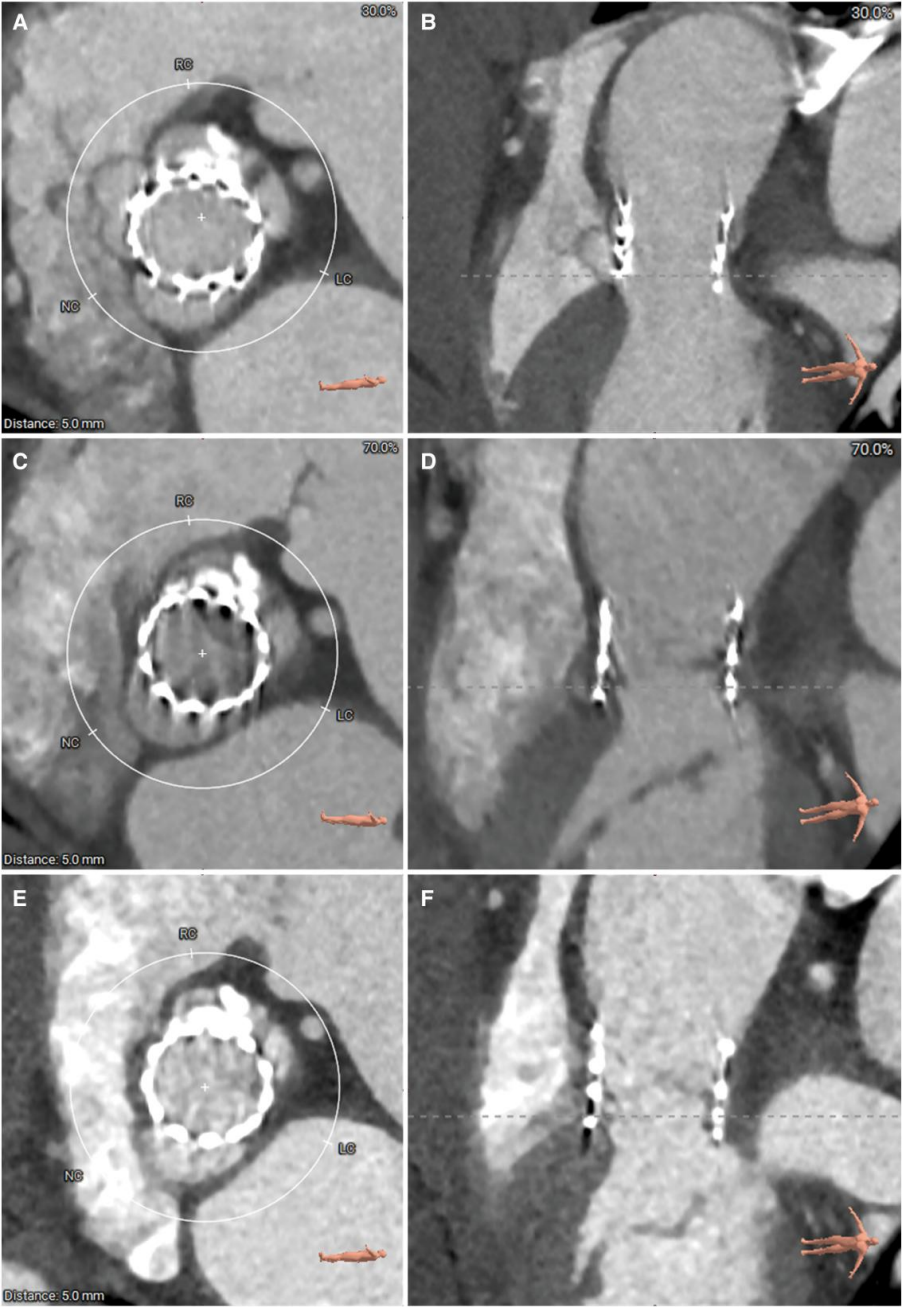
Cardiac computed tomography images of post-procedure, 6-month, and 12-month follow-ups. (*A* and *B*) Computed tomographic images of the interventricular membranous septum aneurysm and in the short view and in the long view post-procedure; (*C* and *D*) computed tomographic images at 6 months; (*E*) and (*F*) computed tomographic images at 12 months.
